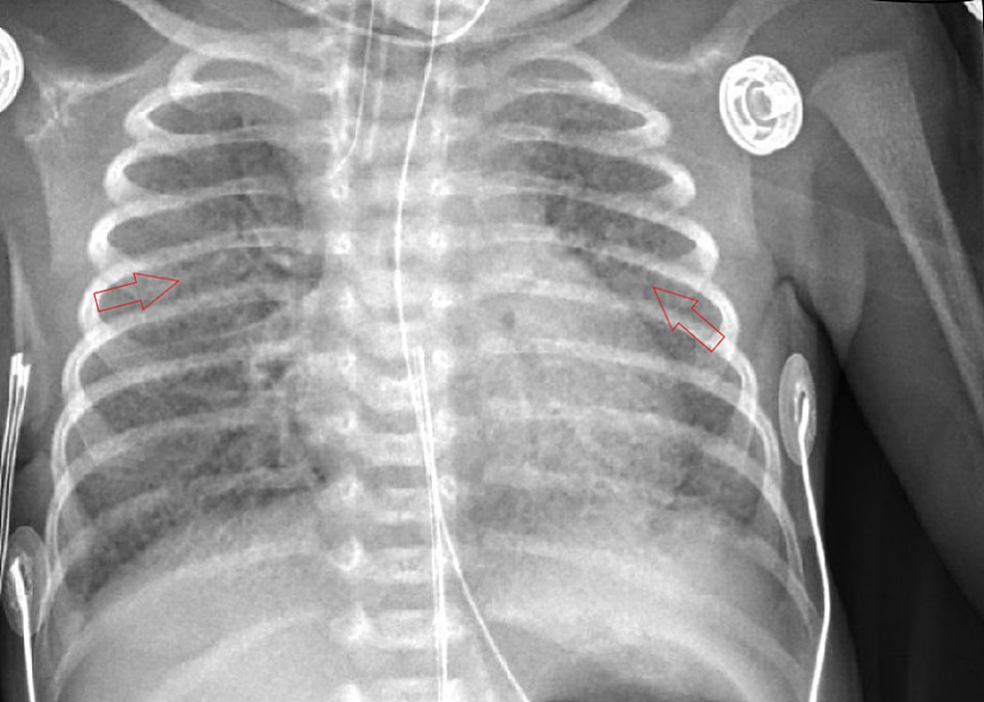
CXR from day 2 of life show diffuse, bilateral, reticulogranular opacification (arrows) CXR - chest x-ray
